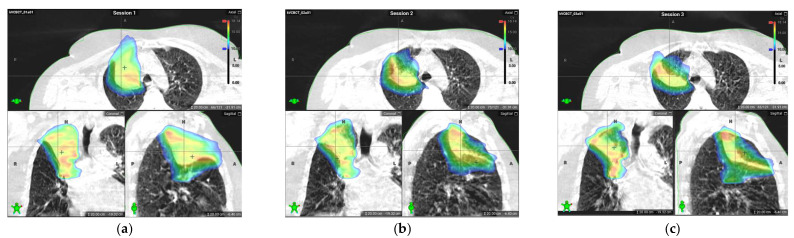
Comparison of dose distributions delivered during the first, second, and third pulses: (a) dose distribution delivered based on cone beam computed tomography (CBCT) images acquired during the first pulse; (b) dose distribution delivered based on the CBCT image acquired during the second pulse, four weeks after first pulse; (c) dose distribution delivered based on the CBCT image acquired during the third pulse, eight weeks after the first pulse. A substantial reduction in lesion size and dose to the surrounding tissue can be seen.
